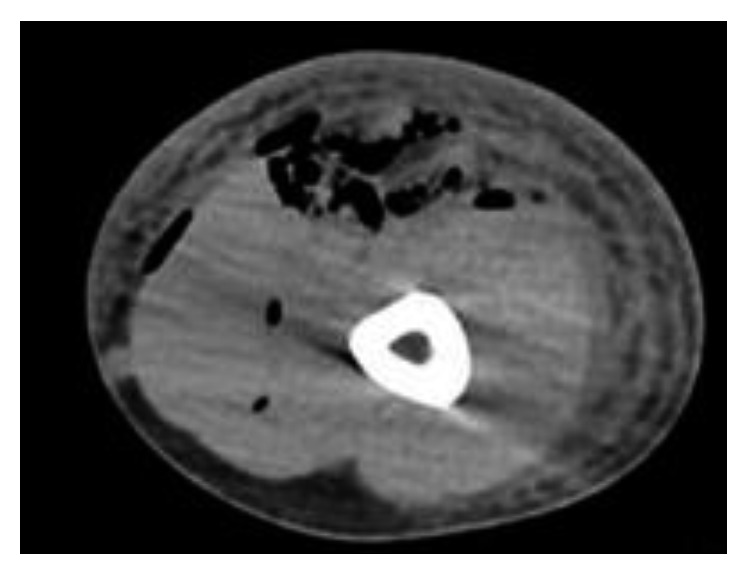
CT scan of the patient's left arm performed at the emergency department showing the presence of subcutaneous emphysema.
